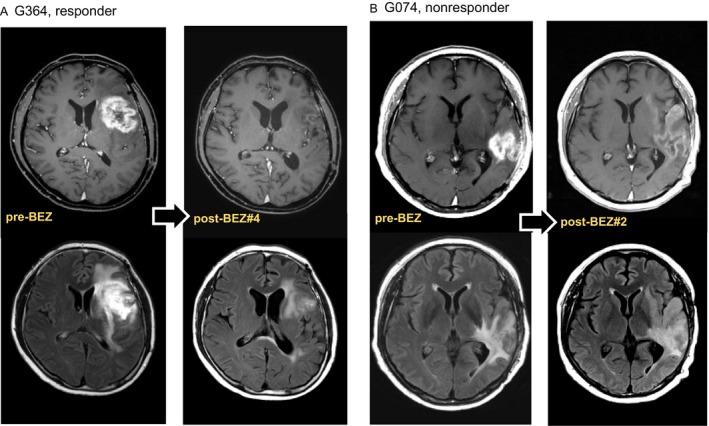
MRIs following BEZ treatment. (A) G364 demonstrated remarkable decrease in contrast enhancement and T2 infiltration accompanied with edema following bevacizumab, while both contrast enhancement and T2 infiltration of G074 (B) had been maintained and somewhat increased despite bevacizumab. MRI, magnetic resonance image; BEZ, bevacizumab; pre‐BEZ, before treatment of bevacizumab; post‐BEZ#2, after 2 cycles of bevacizumab treatment.
